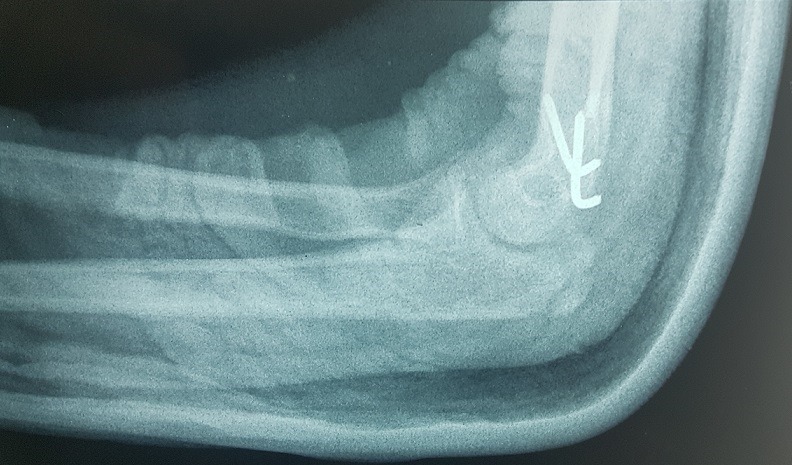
Contrôle radiographique de profil en post opératoire objectivant une bonne reduction
